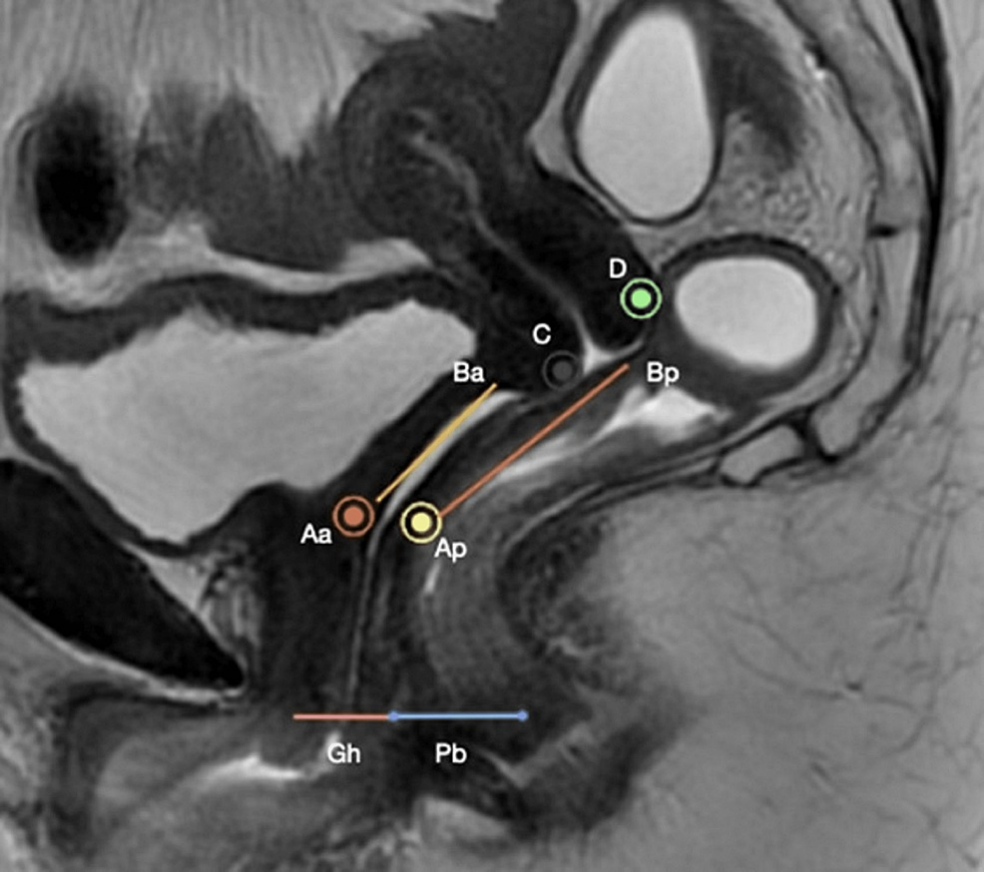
The vaginal topography used in the POP-Q system as depicted on the sagittal T2-weighted image. Note that the bladder is partially filled and the rectum is empty during the POP-Q system. The points are denoted as (+) if they are below the hymen and (-) if they are above the hymen. No sign is assigned to genital hiatus (Gh) and perineal body (Pb), as they are measured independently of the hymen. POP-Q: pelvic organ prolapse quantification.
